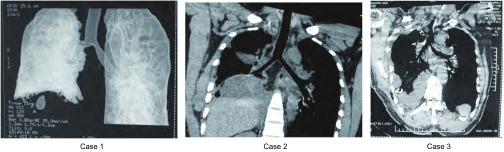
术后2周胸部CT三维重建 The chest CT and bronchial three-dimensional reconstruction on the 14^th^ postoperative day
